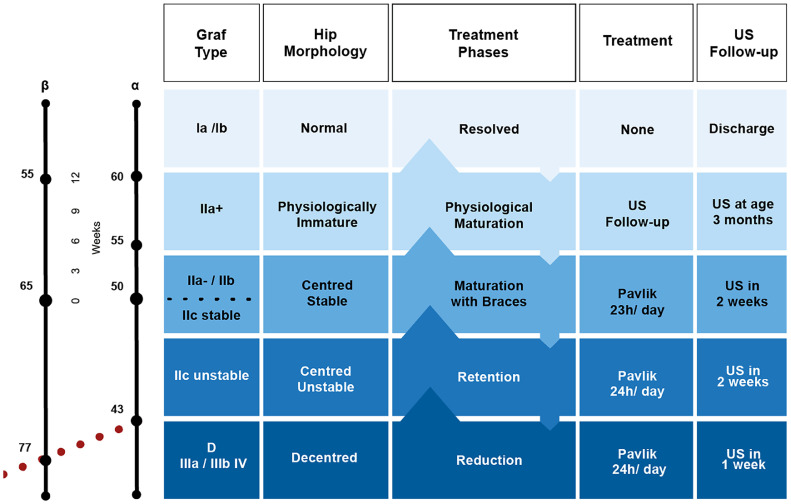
Clinical decision chart for non-operative treatment protocol following the grouping of Graf’s types and subtypes. Graf´s types Ia and Ib are considered normal and do not require treatment. Physiologically immature: Graf type IIa+ are reevaluated at 3-months of age (clinical and ultrasound). Centered stable (Graf type IIa- and IIb, IIc stable), centered unstable (Graf type IIc unstable), decentered (Graf type D/IIIa/IIIb/IV) need treatment. (US, Ultrasound). Dysplastic hips are managed through a structured three-phase protocol reduction, retention, and maturation).
